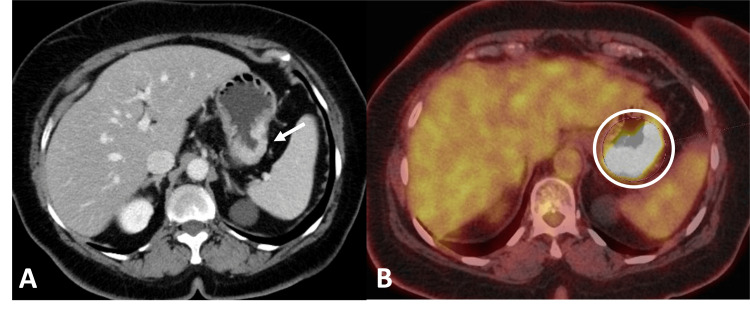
Breast cancer metastasis to the stomach. (A) Axial contrast-enhanced CT showing infiltrative mural thickening and hyperenhancement involving the greater curvature of the stomach (arrow). (B) Axial 18F-FDG PET-CT demonstrating intense FDG uptake (circle) corresponding to the area of gastric wall thickening and hyperenhancement. ^18^F-FDG PET-CT: Fluorine-18 fluorodeoxyglucose positron emission tomography/computed tomography; CT: Computed tomography; FDG: Fluorodeoxyglucose
